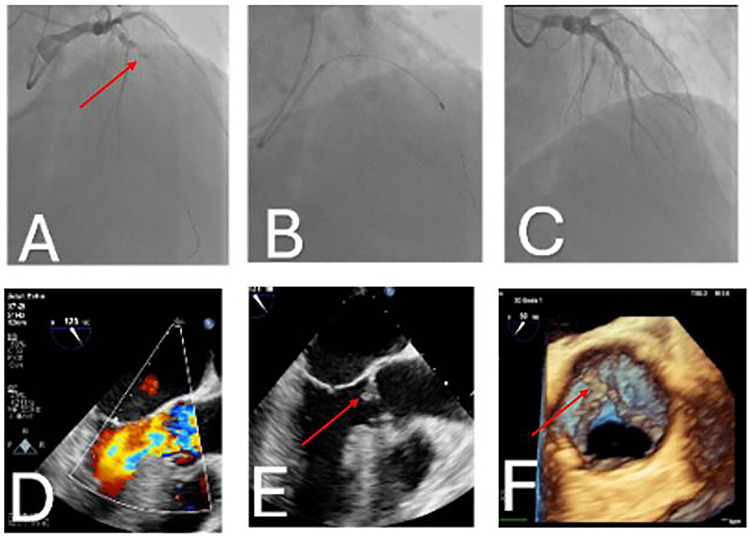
(A) Coronary angiography of the LAD indicating critical stenosis in the mid-portion of the vessel (arrow). (B) Rotablation of an uncrossable lesion in the LAD. (C) LAD after implantation of two DESs. (D) Transesophageal echocardiogram (TEE), color Doppler image showing severe aortic regurgitation. (E) TEE indicating a mobile structure on the non-coronary cusp (arrow). (F) TEE showing a 3D view of the injured non-coronary leaflet (arrow).
